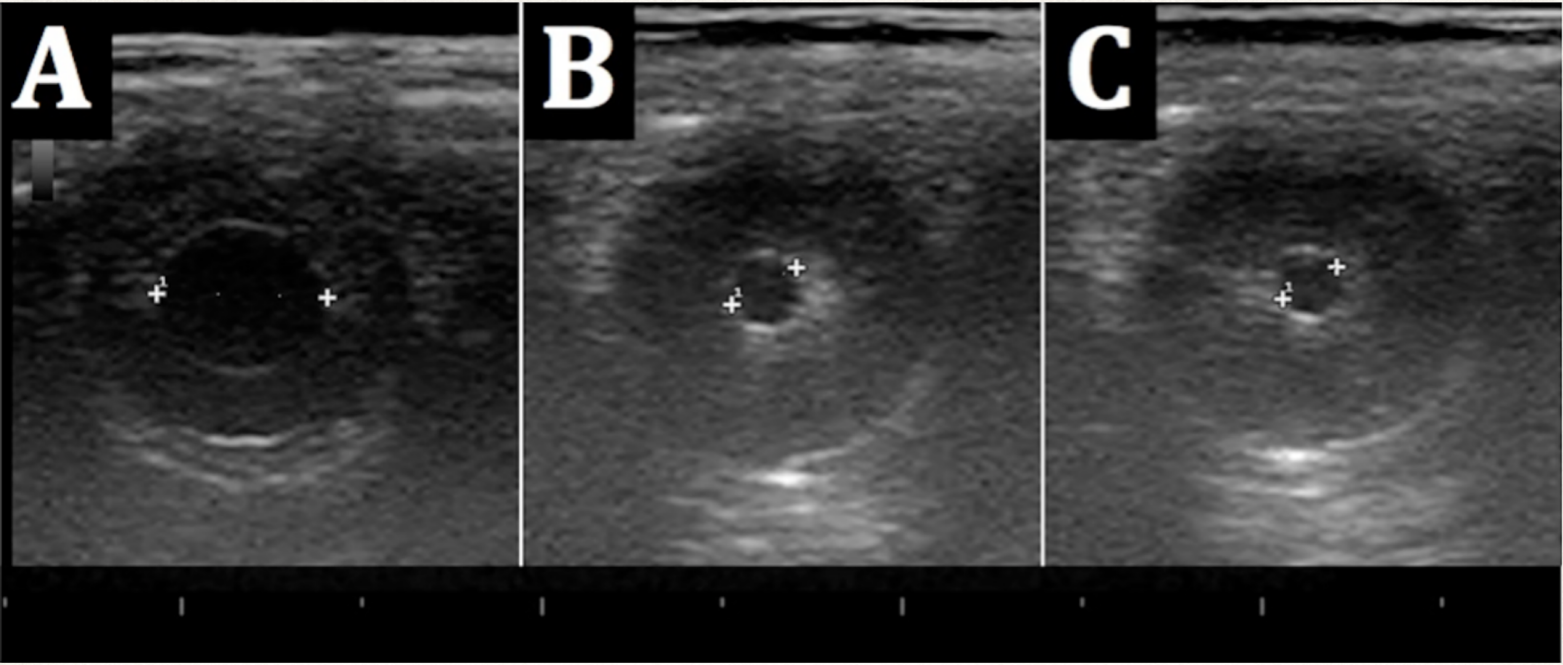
Example of pupillary diameter [PD] assessment in the closed eye by B-mode ultrasound. (A) PD at rest under standard dimmed light conditions of the ultrasound study room. (B) PD during ipsilateral light stimulus [L_stim_]. (C) PD during contralateral L_stim_. Crosses represent the markers set by the examiner for measuring the PD.
